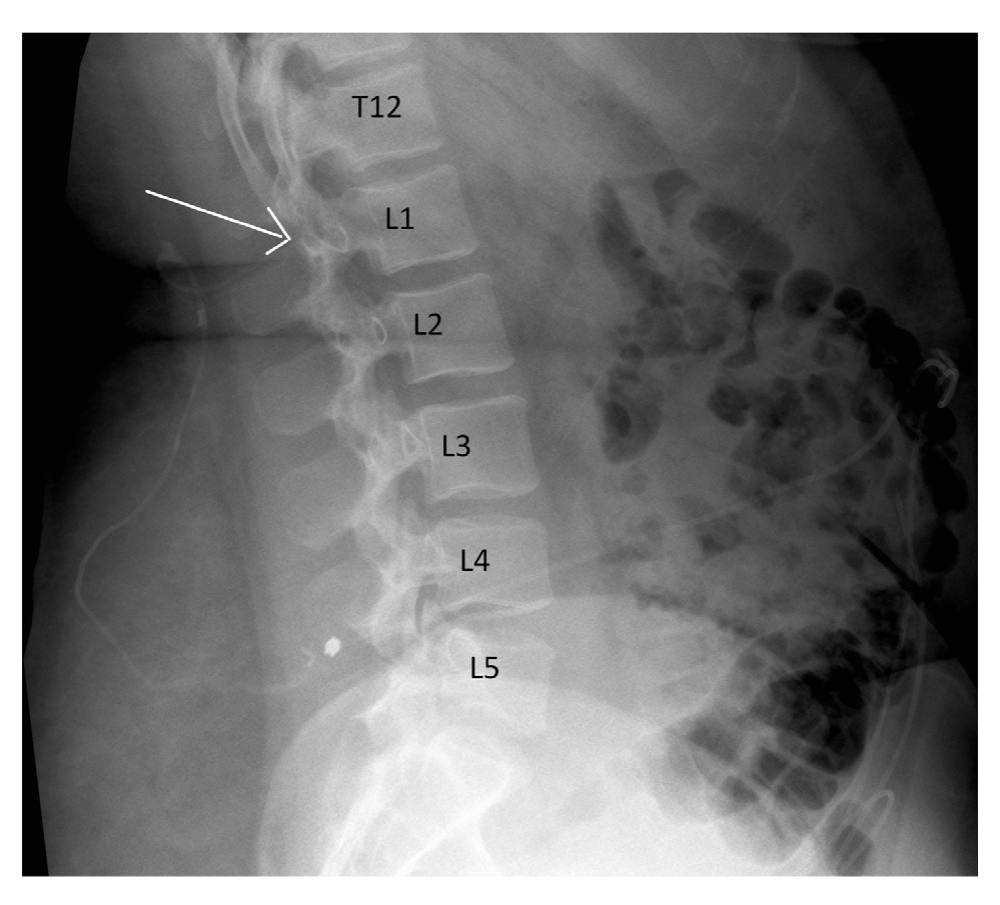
Lateral lumbar x-ray showing incorrect level of lumboperitoneal shunt insertion.
